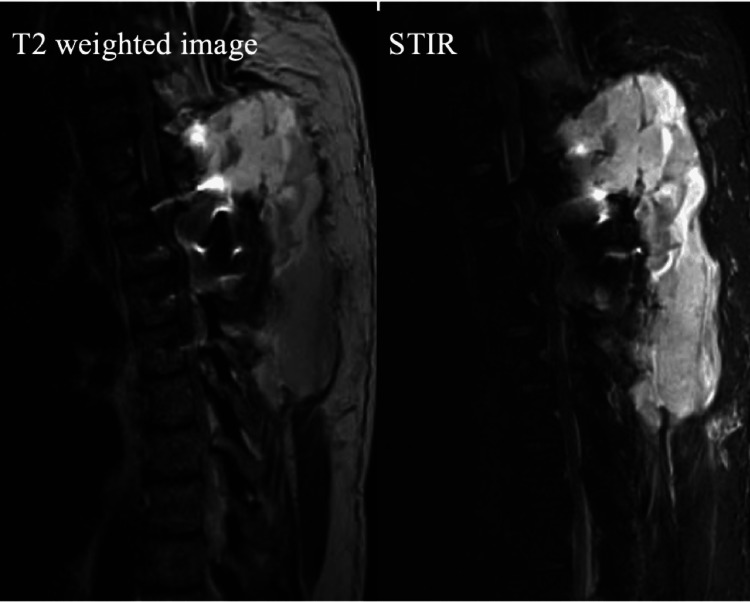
Emergency magnetic resonance imaging of the thoracic spine on postoperative day 12. Extensive fluid accumulation is visualized dorsal to the dura from C7 to T5.
